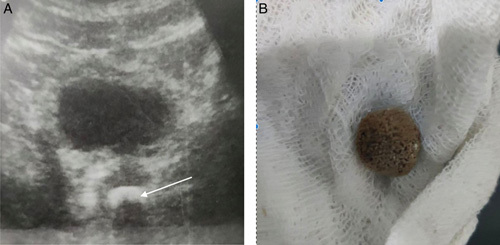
(A) Gray scale ultrasound image showing calculus in the prostatic urethra (white arrow). (B) Image of the urethral stone fragment after extraction.
